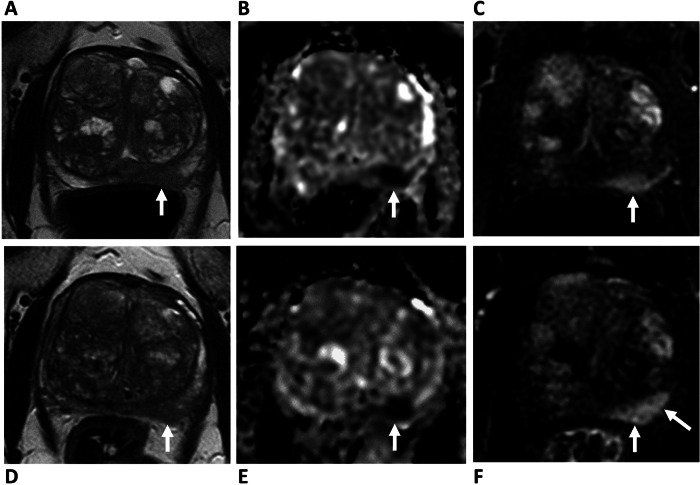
False positive DCE findings on AS follow-up. 74-year-old patient, presenting PSA 10.95 ng/mL. A–C Baseline MRI shows ill-defined T2 change (A) at the left mid-PZ, with a 12 × 9 mm area of marked restricted diffusion (B), with associated focal early enhancement (C), consistent with a PI-RADS 4 lesion (arrows). Targeted biopsy shows Gleason 3 + 4 = 7 (10% Gleason 4) in 3/3 cores. D–F MRI at 12 months, PSA 10.84 ng/mL. Stable conspicuity and size of lesion on T2 (D) and ADC maps (E), but increase in the degree of enhancement to 22 × 12 mm (F, arrows). PRECISE score 3—findings considered a false positive. The patient remains on AS
